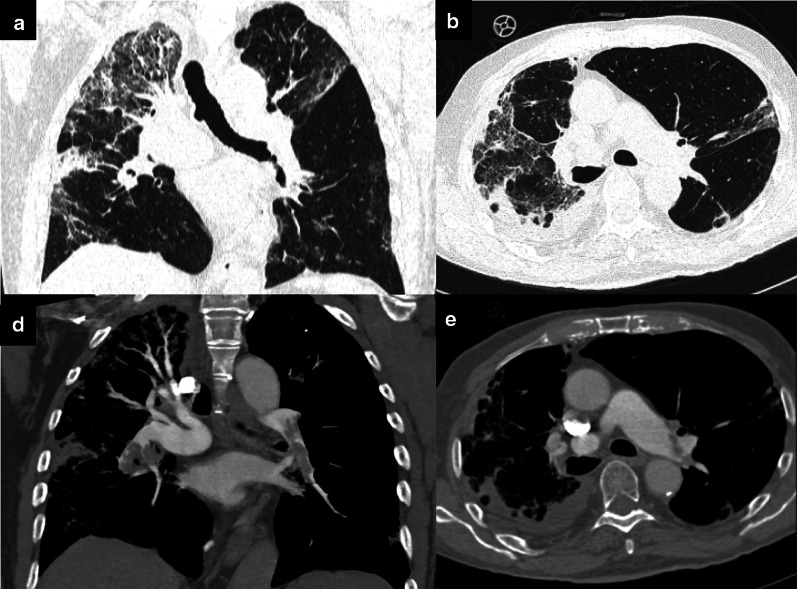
Pulmonary Embolism. COVID-19 patient with dyspnea, increased D-dimer levels and O2 saturation < 93. Chest CT evidenced GGO with interstitial thickening of the interlobular and intralobular septa, together with pleural effusion (a, b). After contrast media injection, PE was found in the left upper lobar branch, the left inferior lobar branch and the segmental arteries for the left inferior pulmonary lobe and PE of the interlobar arteries for the inferior right lobe, probably extended to the subsegmentary arteries (d, e). *CT* computed tomography; *GGO* ground glass opacity; *PE* pulmonary embolism
